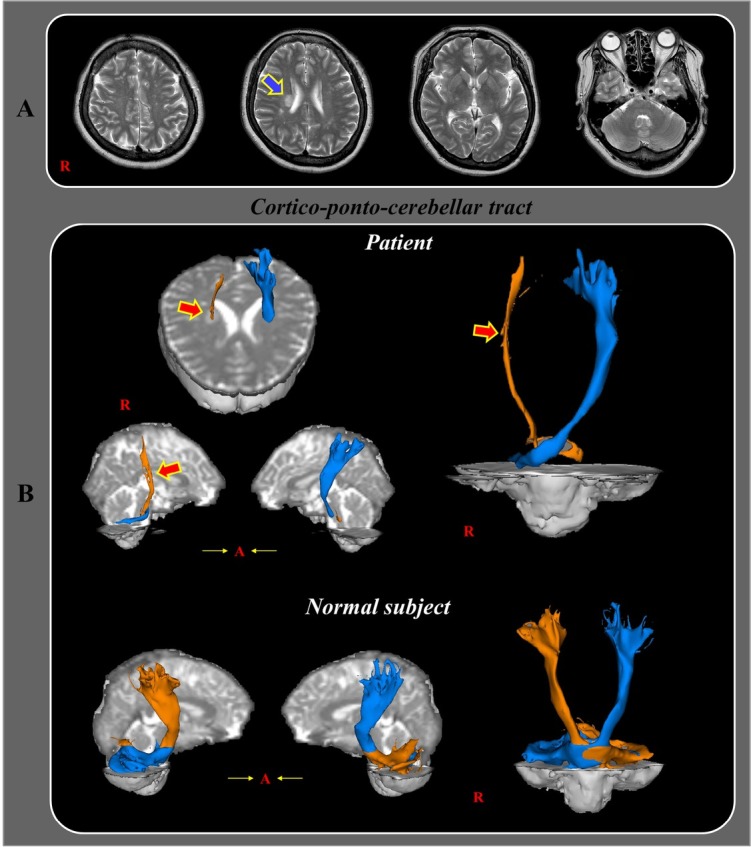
(A) T2-weighted brain magnetic resonance images taken 2 weeks after disease onset showing a right corona radiata infarct (blue arrow). (B) Diffusion tensor tractography in the cortico-ponto-cerebellar tract (CPCT). Thinning of the right CPCT is visible (red arrows). The left CPCT is well-preserved. The bilateral CPCT images in a healthy control (50-year-old male) without any injury are presented.
